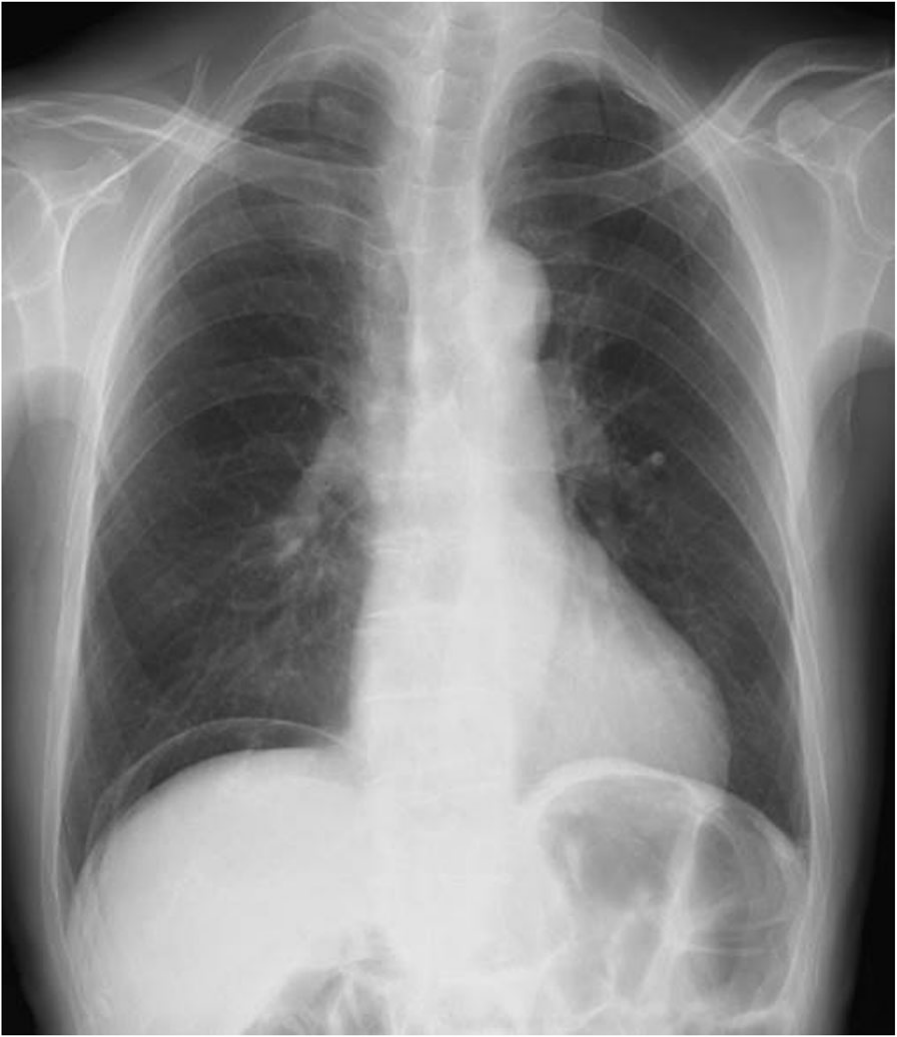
Chest radiograph showing free gas in the right subdiaphragmatic region
